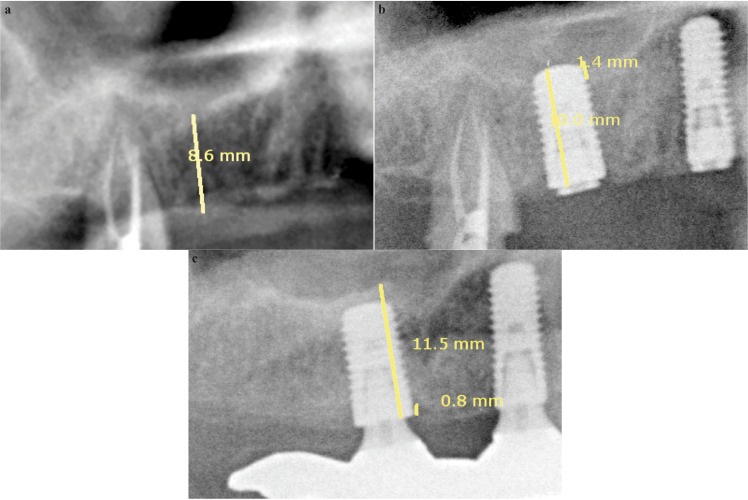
Radiographic evolution of sinus demarcation: a) before surgery, b) Year 1 and c) Year 2.
